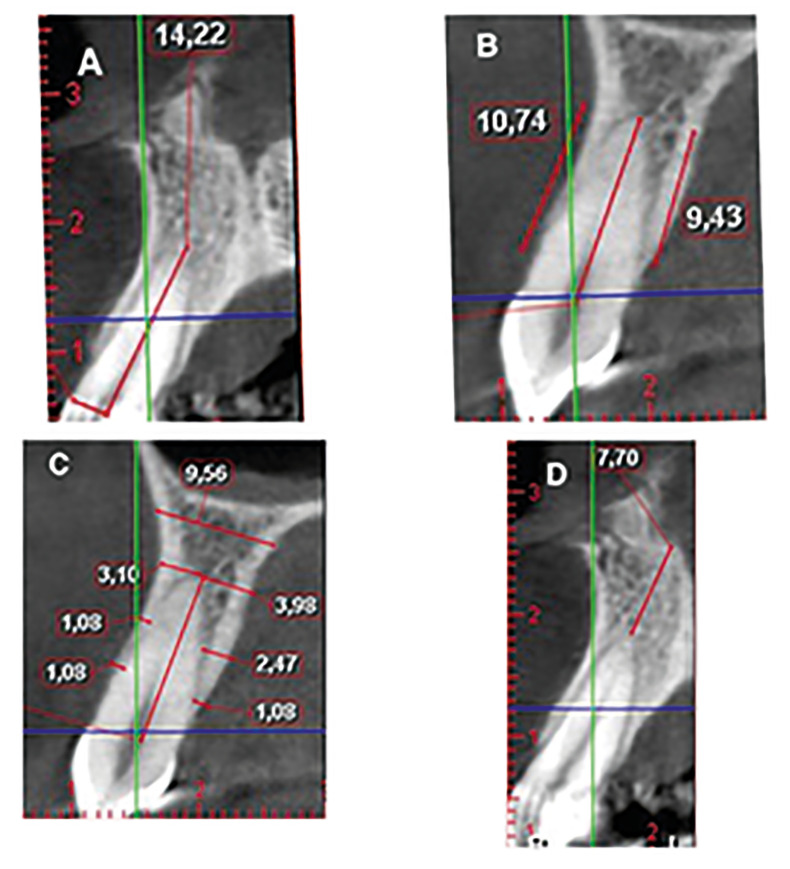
Measurements taken on every CBCT image. (A) Method of measuring the root length. Measurement of the distance from the CEJ projected on the midpoint of the root on a sagittal vision, to the point of the root apex. (B) Method of measuring the buccal and palatal bone height.A line is drawn from the most coronal point (bone peak) of the buccal and palatal cortical, to the point of the root apex on both buccal and palatal sides. (C) Method of measuring the thickness of the buccal and palatal corticals. Three lines were drawn perpendicular to the sagittal axis of the root, both buccal and palatal: line A: 1mm apical to the alveolar crest, line C: at the apical point, and line B: in the middle distance between points A and C. At 4mm apical from the root apex, the post-apical line is drawn from buccal to palatal sides. (D) Method of measuring the apical bone height. A line is drawn from the apex of the tooth to the floor of the nostril.
